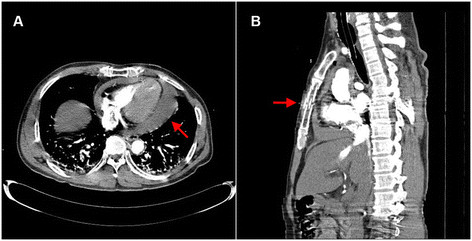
Chest computed tomography at admission. (A) The hemopericardium (red arrow) in the transverse view. (B) The sternal fracture (red arrow) in the saggital view.
